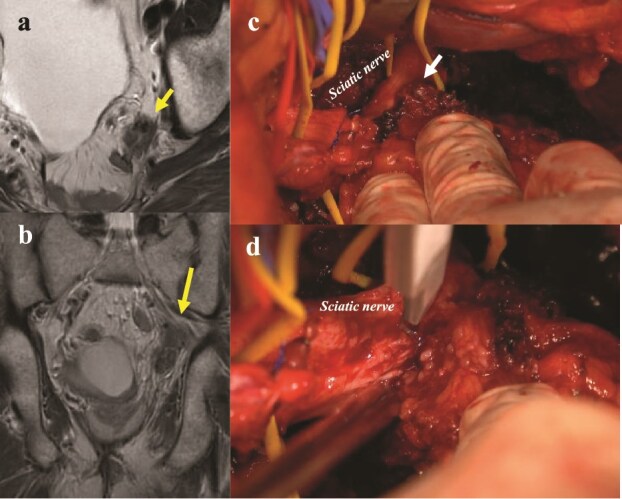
Pelvic sidewall exenteration (partial resection of the sciatic nerve). (a, b) Preoperative MRI images indicate suspected invasion of the sciatic nerve by the recurrent tumor (arrows). (c) Intraoperative photograph shows direct tumor infiltration into the sciatic nerve (arrow). (d) Partial resection of the sciatic nerve performed using a scalpel. R0 resection was achieved pathologically. Six months postoperatively, the patient is undergoing rehabilitation; walking with a cane after initial recovery with a walker.
